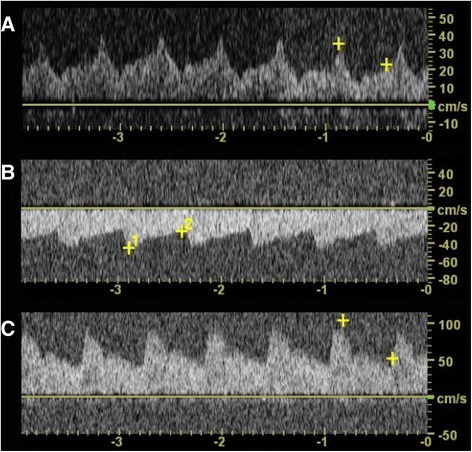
Transcranial doppler flow patterns. Sequential transcranial doppler (TCD) flow patterns are displayed. The left MCA is imaged via temporal bone window at a depth of approximately 5 cm. Panel a shows anterograde flow through the left MCA with the patient manually occluding the bypass graft (flow pattern *above* the line). The significance of the wave form is uncertain. Panel b display the retrograde flow pattern in the left MCA delivered through a patent EC-IC bypass graft (flow pattern *below* the line). In panel c is TCD done over the contralateral MCA showing a normal flow pattern
